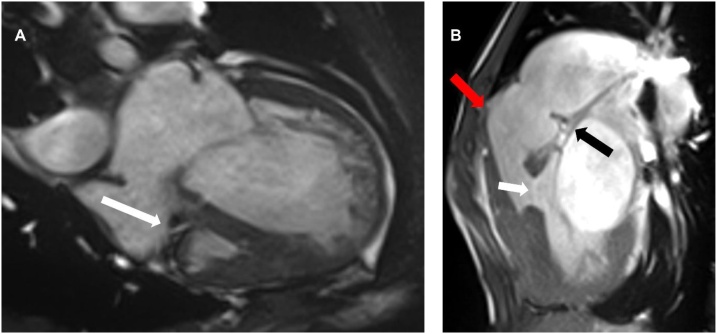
Cardiac magnetic resonance imaging. A) Four-chamber view with visualization of the atretric tricuspid valve (arrow) and the residual right ventricle. B) Sagittal view with visualization of the non-restrictive ventricular septal defect (white arrow), the pulmonary valve (red arrow), and the residual non-restrictive pulmonary banding (black arrow).
